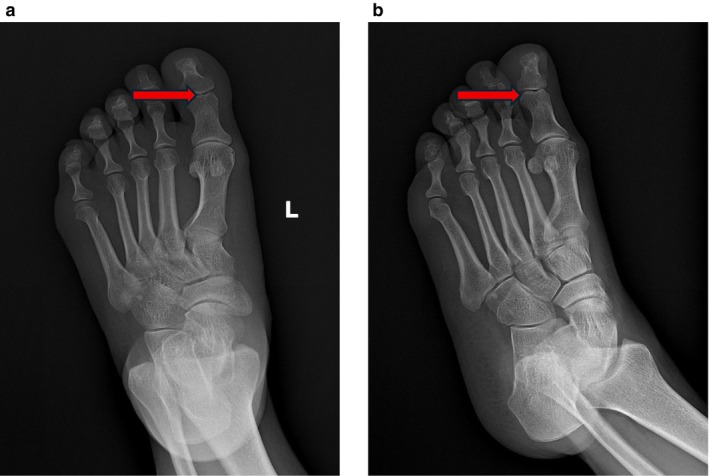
Preoperative radiograph of the left foot demonstrates the presence of a sesamoid bone within the interphalangeal joint. The structure indicated by the red arrow corresponds to this sesamoid bone. Based on these findings, the patient is considered to have congenital sesamoid impaction.
